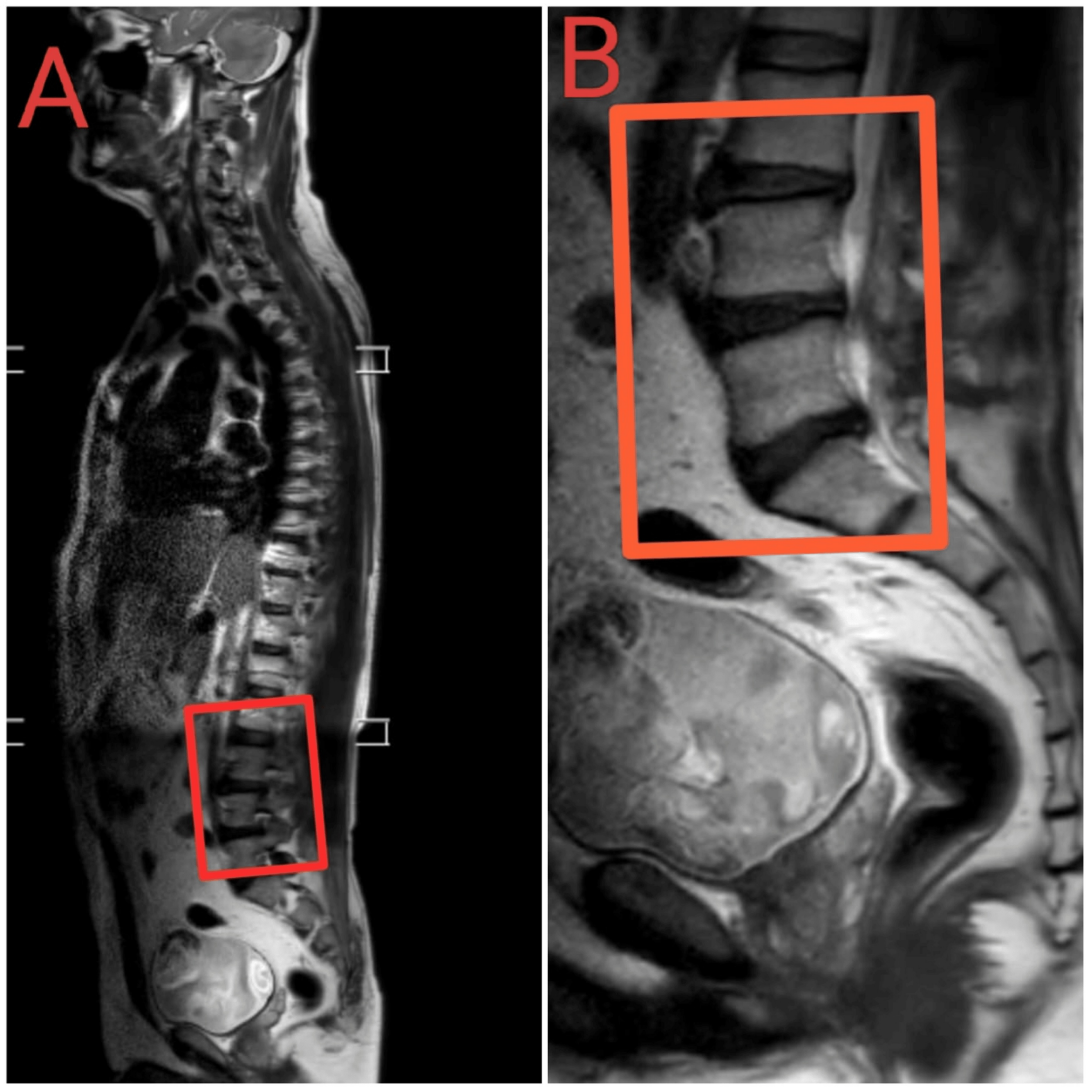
Presurgical magnetic resonance image This presurgery MRI image depicts sagittal views of the lumbar spine. The right image (A) shows the full length of the spine, while the left (B) image focuses on the lower lumbar region, specifically highlighting the L4-L5 and L5-S1 intervertebral discs
